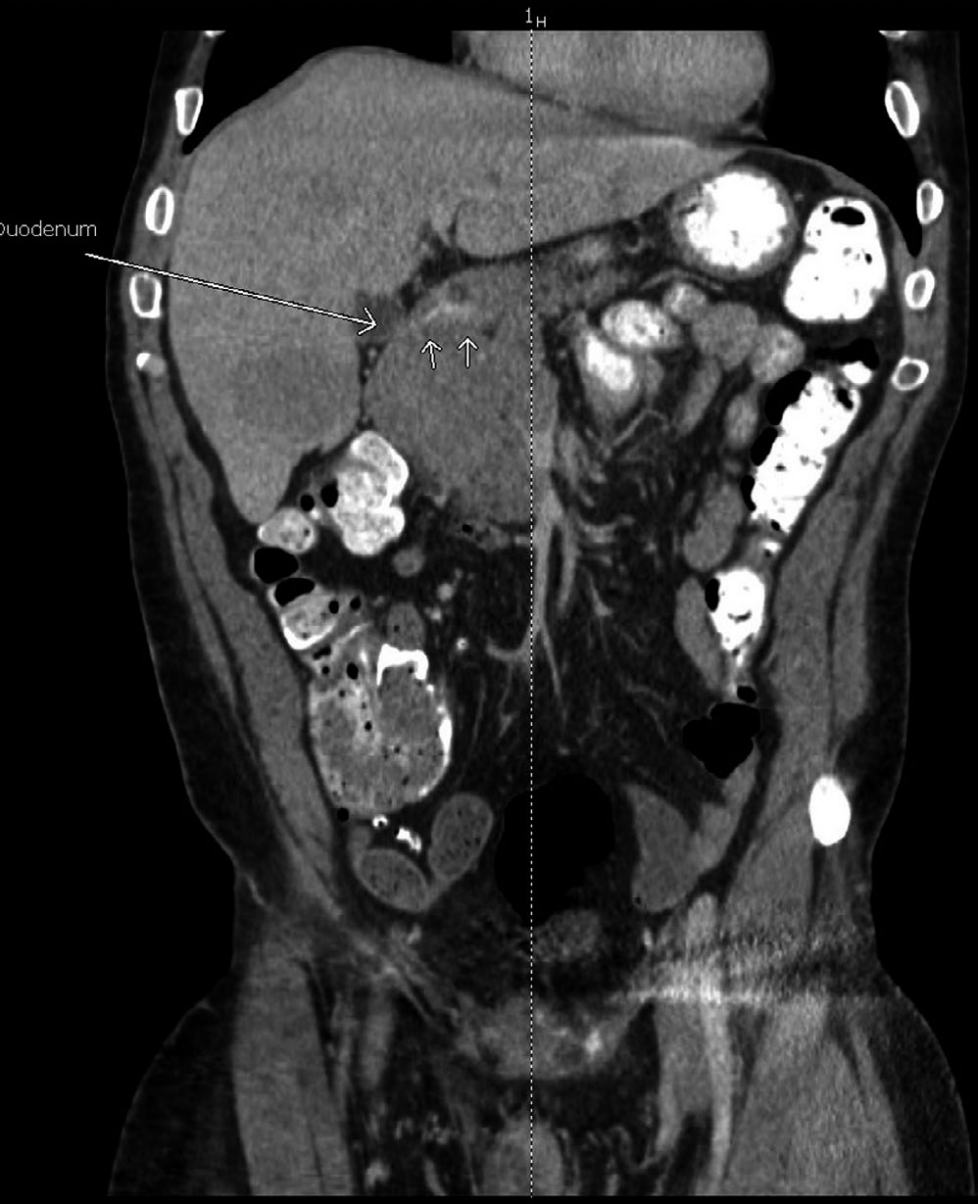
Coronal and axial cuts of an abdominal CT demonstrating pancreatic mass with duodenal invasion
